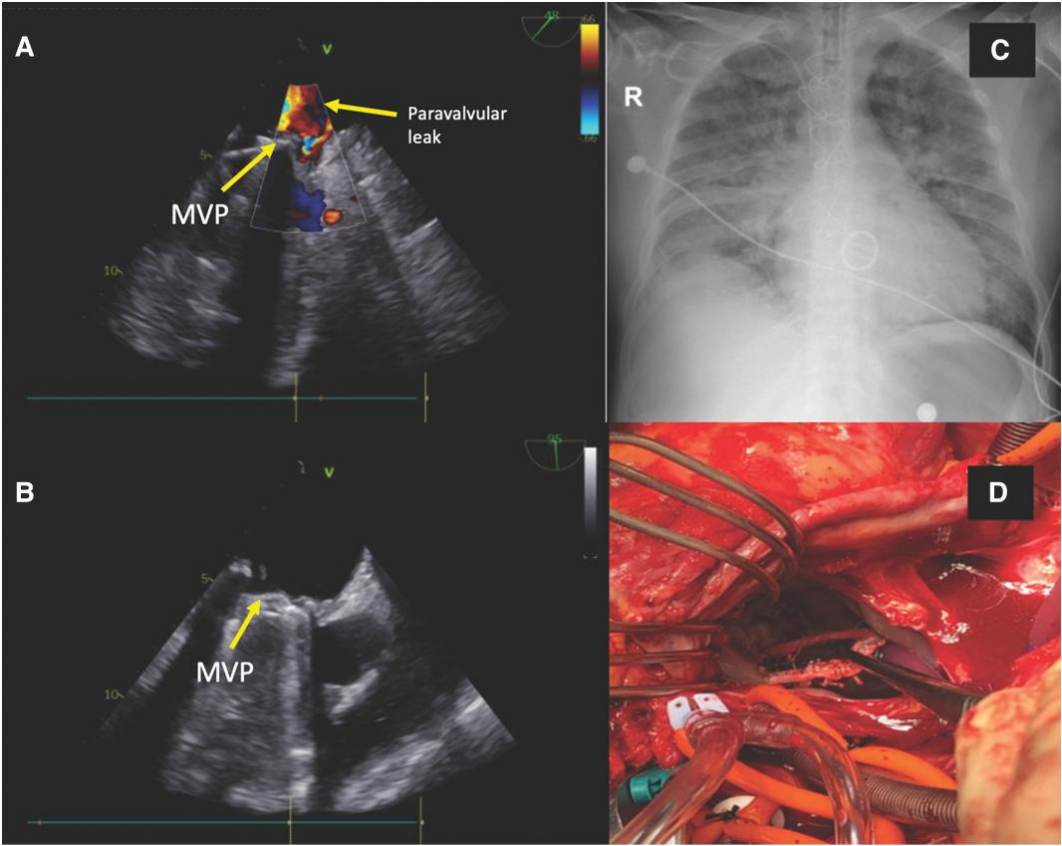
Case 2: (*A* and *B*) Transoesophageal echocardiogram in mid-oesophageal projection at the level of the mitral prosthesis. Doppler (*A*) shows moderate paravalvular leak due to detachment of the posterior mitral ring. (*B*) Two-dimensional model shows opening and closing of the mitral valve discs. (*C*) Increase in size of pulmonary hilum due to pre-capillary pulmonary hypertension, pulmonary oedema with bilateral central predominance opacities, and right pleural effusion or thickening. (*D*) Atrial septal approach for mitral valve replacement: mechanical mitral valve dehiscence due to endocarditis leading to shock.
